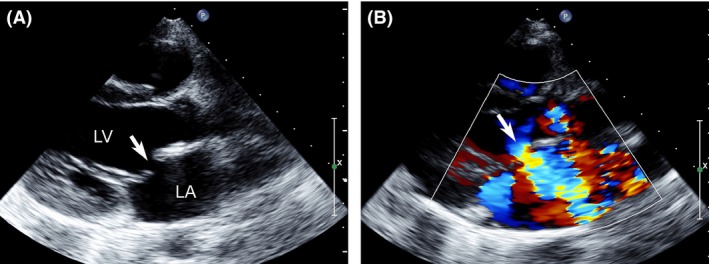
Parasternal left ventricular long‐axis view: Two‐dimensional transthoracic echocardiography showed anterior mitral leaflet perforation (arrow). Parasternal left ventricular long‐axis view: Two‐dimensional color Doppler imaging showed severe mitral regurgitation (arrow). LA, left atrium; LV, left ventricle.
